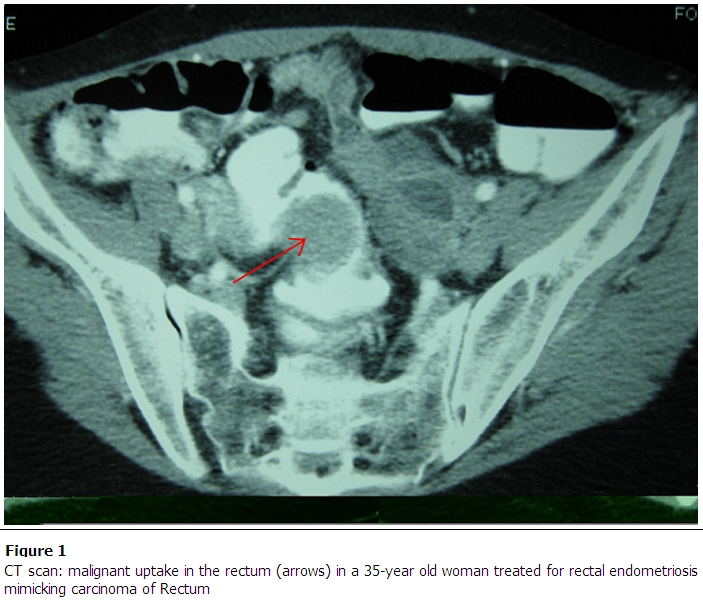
CT scan: malignant uptake in the rectum (arrows) in a 35-year old woman treated for rectal endometriosis mimicking carcinoma of Rectum
